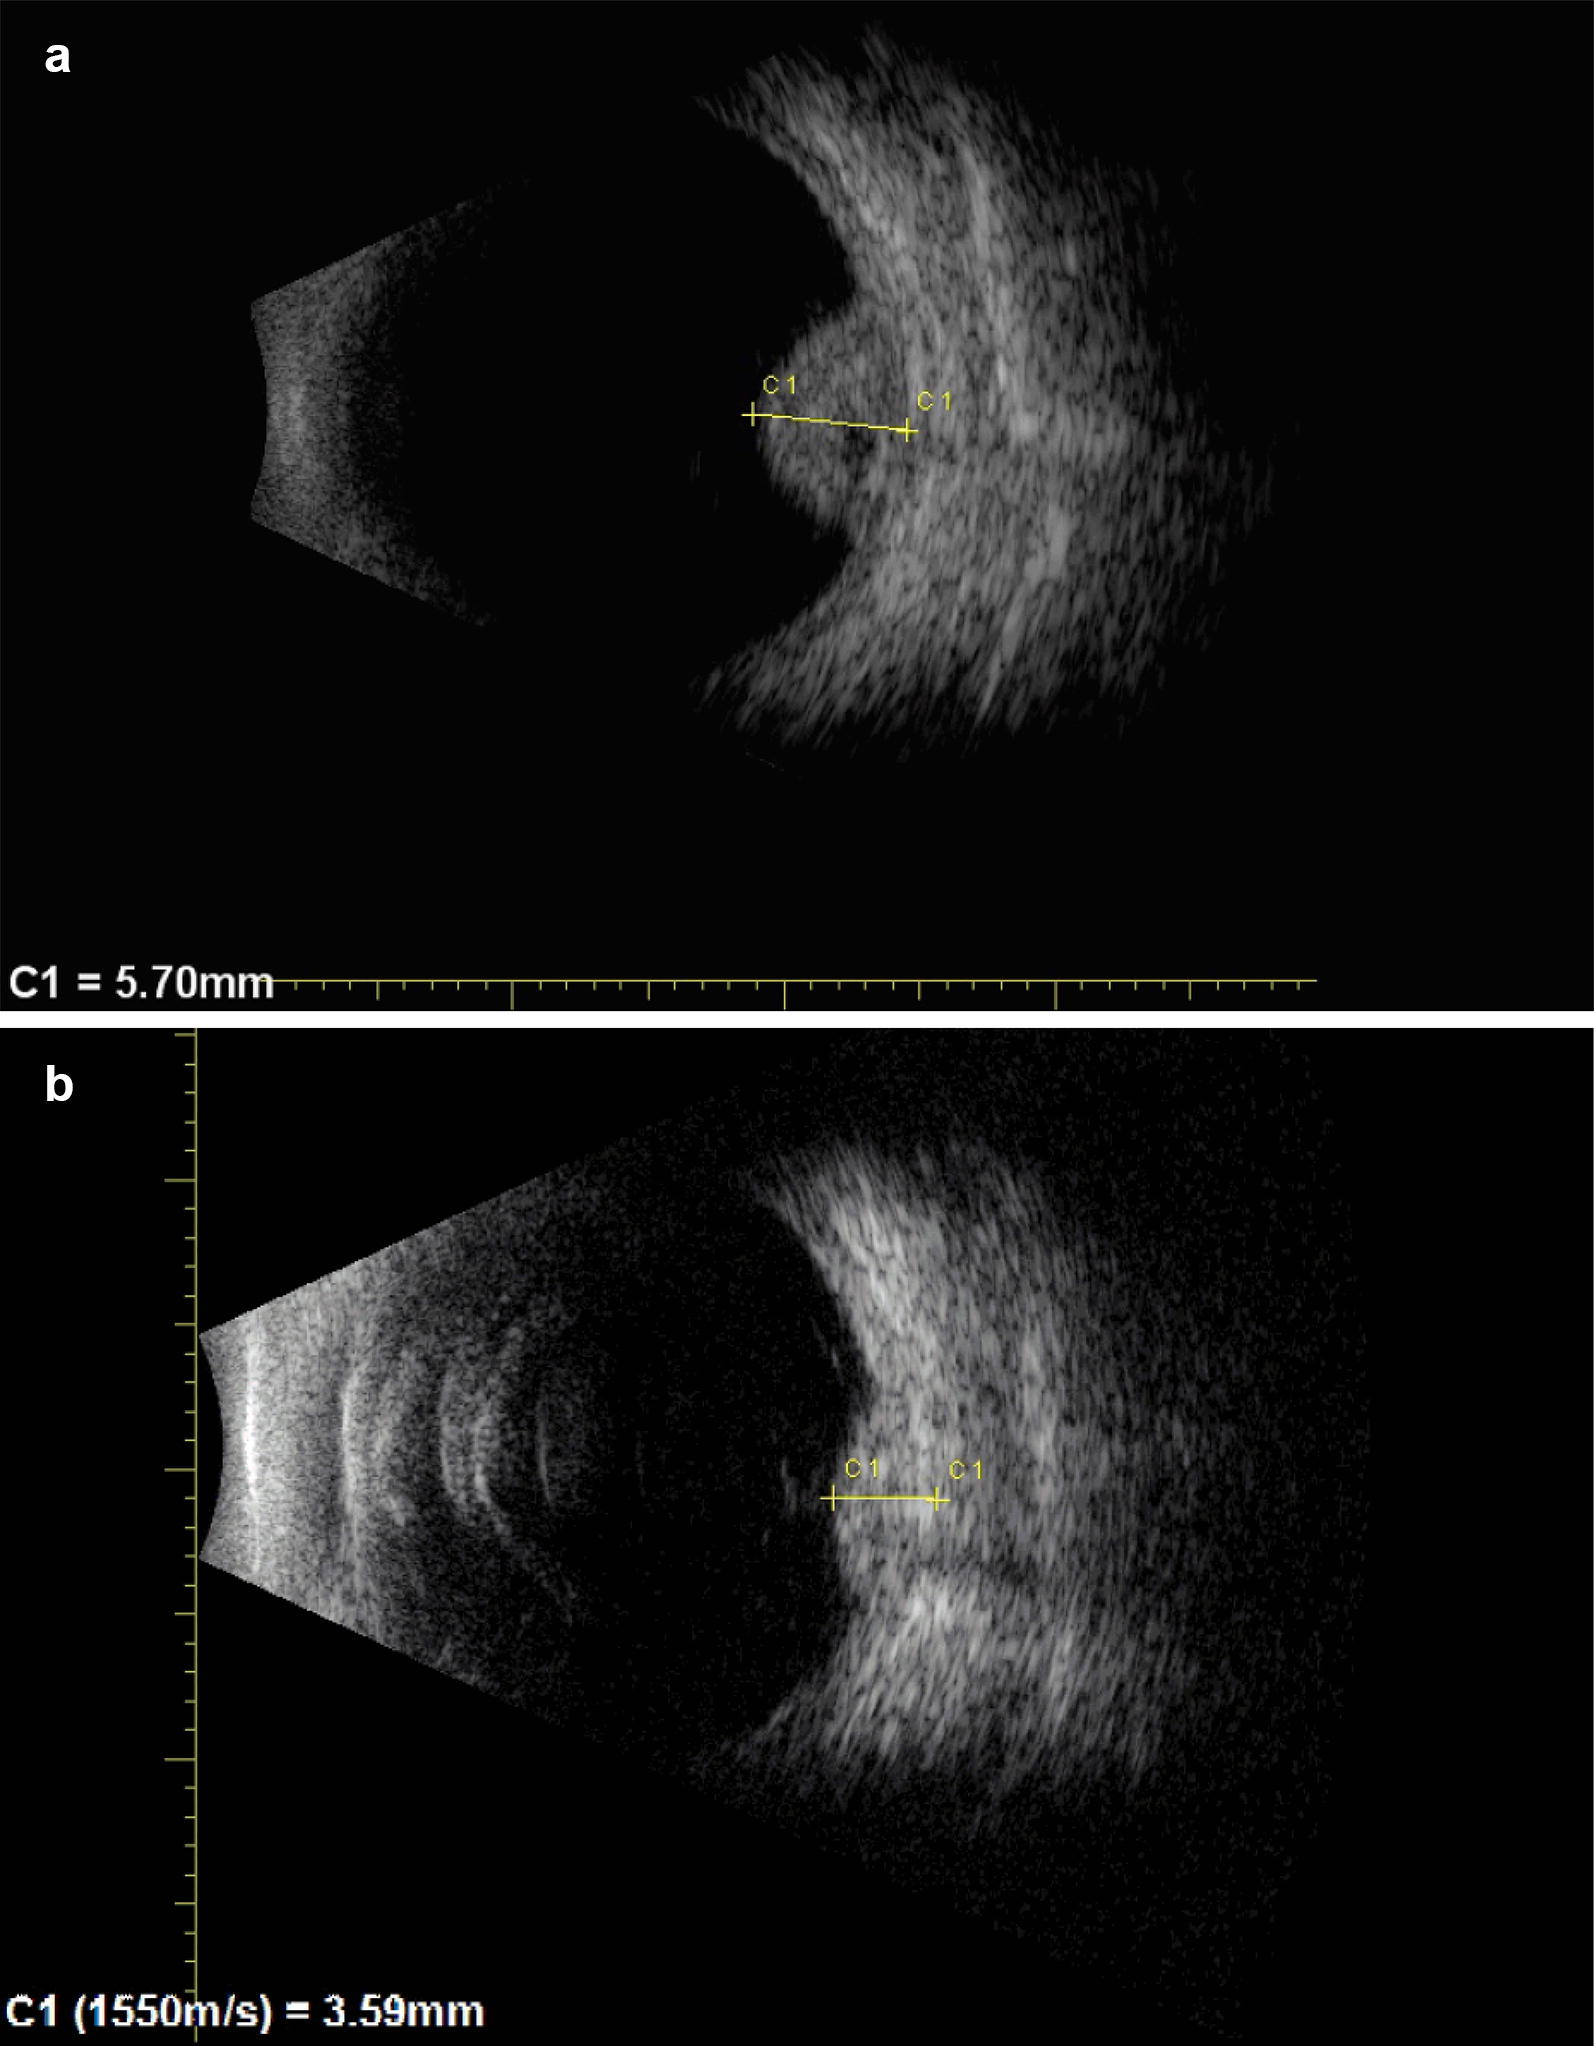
Ultrasonography of the patient a immediately before GK radiosurgery: tumor has increased in thickness to 5.7 mm after years of stabilization, and b in October 2017 the tumor has successfully shrunk after GKR to be 3.59 mm
